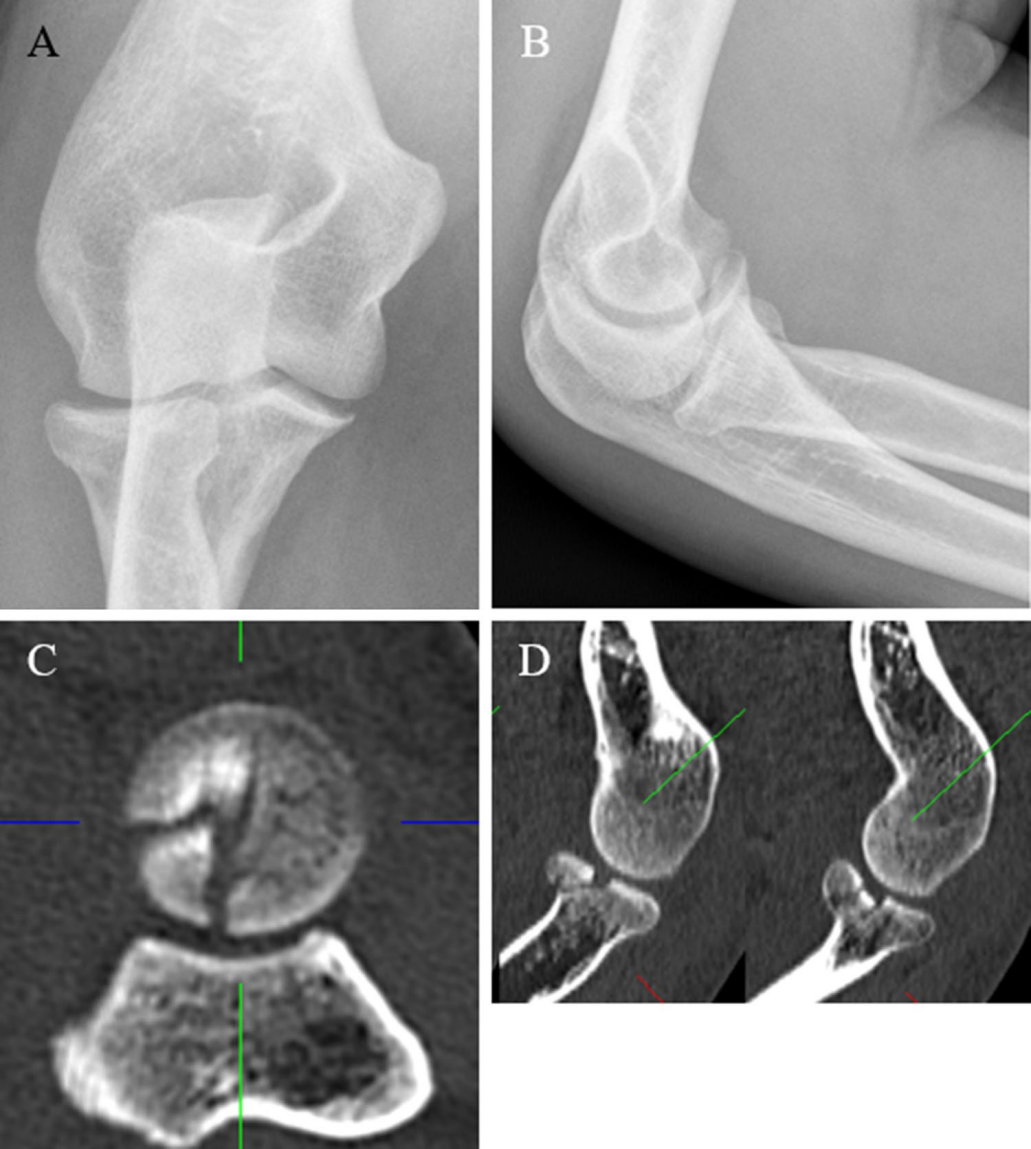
a, b Preoperative radiographs (anteroposterior and lateral) of a Mason type III fracture (Case 2). c CT-scan in axial view and d in sagittal view
